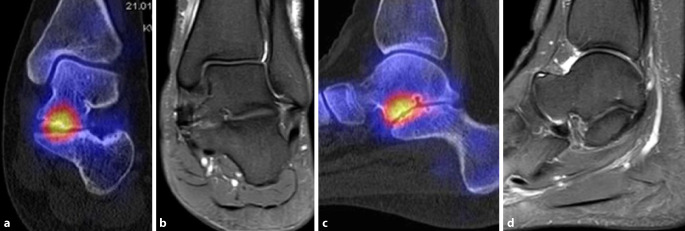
Arthrodesen gehören am Fuß zu den etablierten Therapieverfahren bei schmerzhaften Arthrosen. Eine zumindest teilweise knöcherne Durchbauung von Arthrodesen sollte in der Regel innerhalb von 6 Monaten postoperativ nachweisbar sein. Eine unvollständige oder fehlende Fusion nach Arthrodese kann über eine permanente Stressreaktion ähnlich wie bei Koalitiones persistierende Schmerzen verursachen, was sich mit der SPECT/CT nachweisen lässt. Studien zur Dauer einer erhöhten Osteoblastenaktivität nach Arthrodesen sind nicht publiziert. Unseren Erfahrungen nach ist 6–9 Monate nach Arthrodesenoperation häufig noch ein deutlich erhöhter Stoffwechsel bei nachweisbarer Durchbauung der Arthrodese zu sehen, weil die Osteoblasten noch aktiv sind; nach 1–1,5 Jahren sollte die Intensität der Anreicherung deutlich abgeklungen bzw. nur noch gering sein. Der Vorteil der SPECT/CT gegenüber der alleinigen CT bei persistierenden Beschwerden nach Arthrodesen ist, dass sowohl die CT-Morphologie als auch die Osteoblastenaktivität um die Arthrodese und in den Nachbargelenken untersucht wird. Somit können symptomatische Anschlussarthrosen nachgewiesen oder ausgeschlossen werden.
